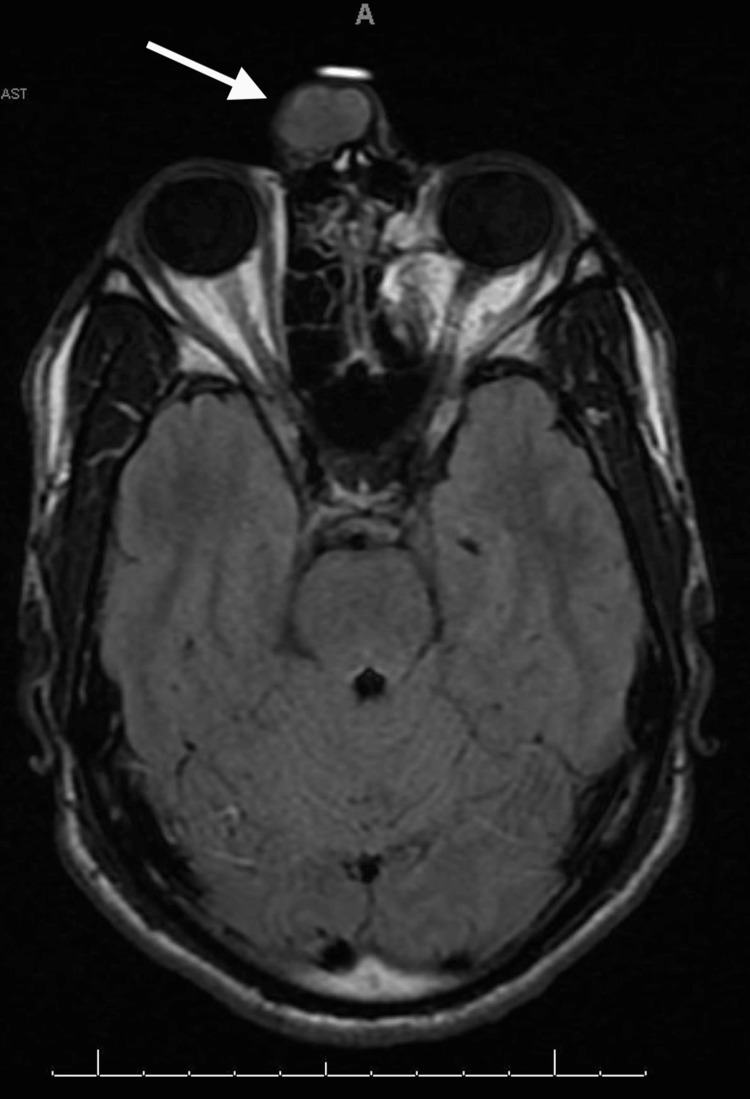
Preoperative MRI (pre-contrast T1-weighted) Preoperative MRI: axial cut of a pre-contrast T1-weighted image with an arrow demonstrating a hypointense mass within the glabellar region MRI: Magnetic resonance imaging
